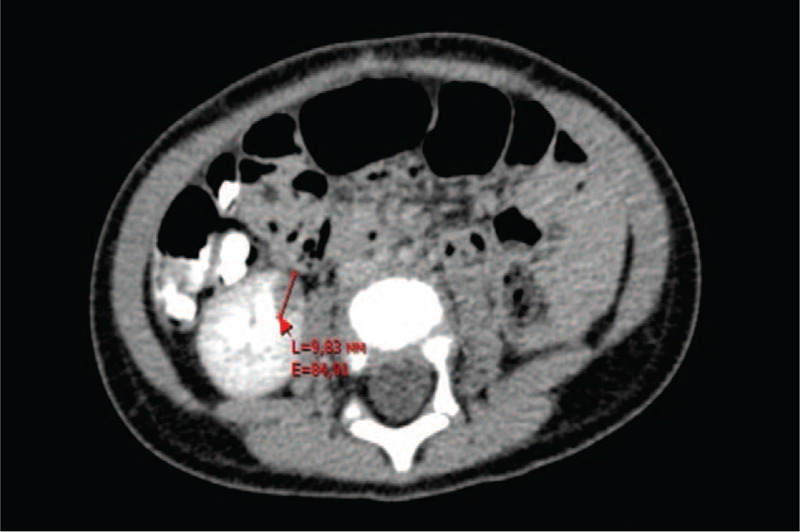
Contrast-enhanced CT scan obtained 8 months after tumor nephrectomy of left kidney showing an oval hypodense lesion at the medial aspect of the right kidney with a maximum diameter of 10 mm.
